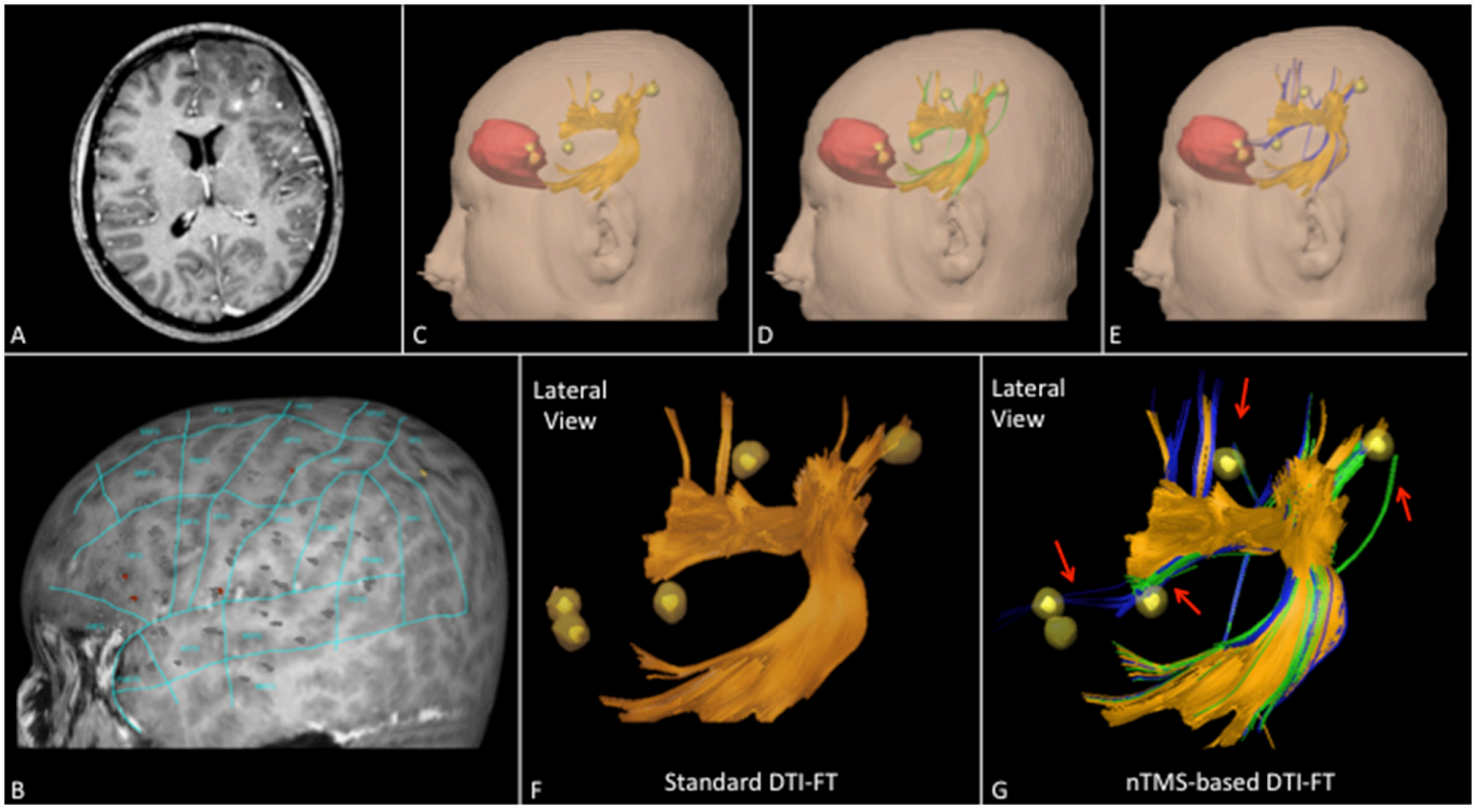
(A) T1-weighted contrast enhanced sequence showing a case of a left frontal anaplastic astrocytoma. (B) nTMS language mapping findings according to Corina's cortical parcellation system (Corina et al., 2005, 2010). (C) Lateral view of AF (orange) computed by using the standard DTI-FT technique showing its spatial relationship with nTMS spots (yellow) and tumor (red). (D) Lateral view of AF computed by using the nTMS-based “all-spots”, and (E) “single-spot” strategy showing new additional fibers (respectively green and blue) connecting AF to nTMS spots. (F) 3D reconstruction of AF by using standard and (G) nTMS-based DTI-FT. It's interesting to note the additional fibers obtained by using the “all-spots” and the “single-spot” strategy connecting AF to cortical nTMS spots (red arrows).
